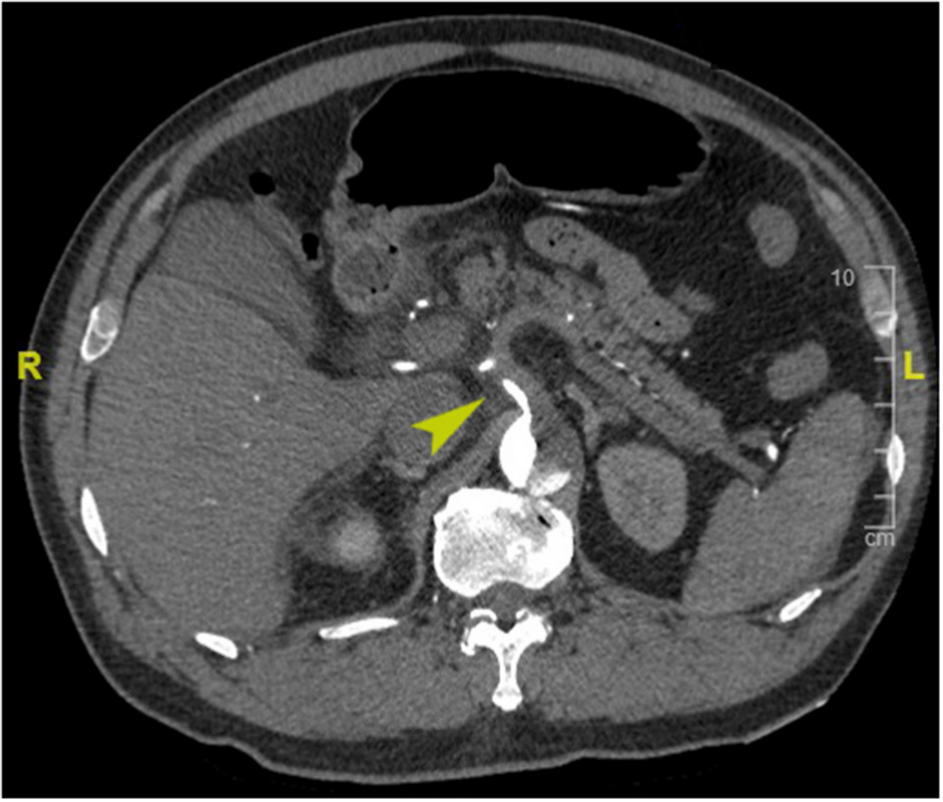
Enhanced computed tomography angiography image of a representative case of preoperative celiac trunk malperfusion (arrowhead).
